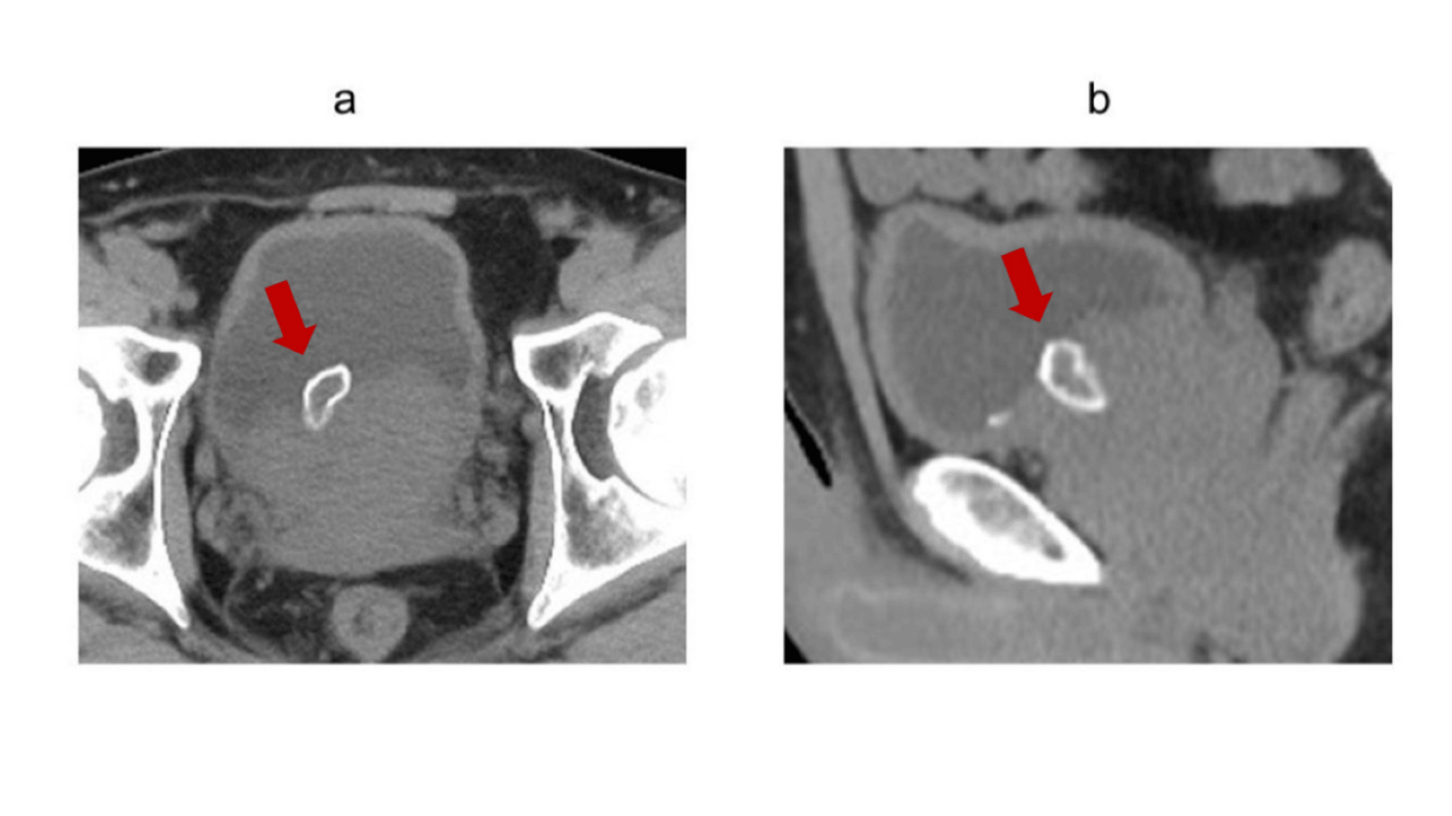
Computed tomography findings Computed tomography demonstrated a soft tissue mass with surface calcification obstructing the bladder neck. a) Axial image. b) Sagittal image.
